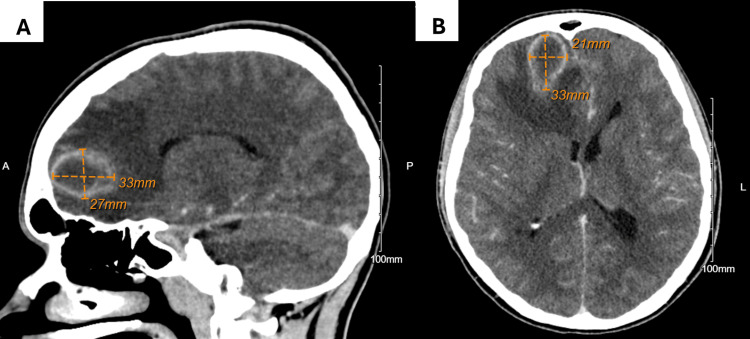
CT scan of the brain demonstrating the right frontal lobe abscess The sagittal view (A) and axial view (B) of the brain CT scan with and without contrast on admission, revealing a 3.3 cm x 2.1 cm x 2.7 cm right frontal lobe abscess.
